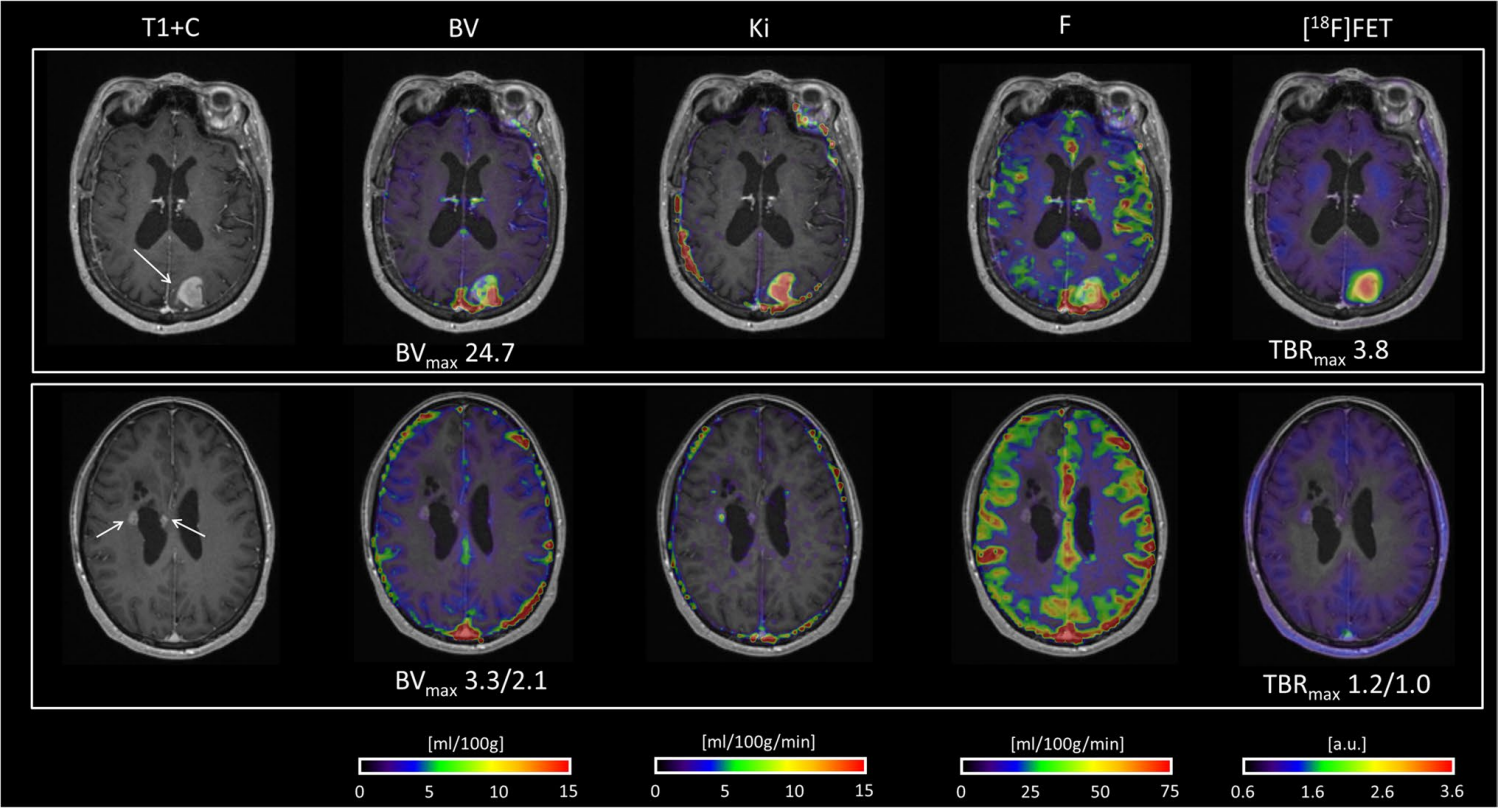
Examples of [^18^F]FET PET and DCE BV imaging providing similar information. *Upper row:* IDH-mutated GBM, progressive enhancing lesion in the left occipital lobe 7 months after surgery and radiotherapy and 1 month after completing adjuvant temozolomide. Subsequent surgery confirmed recurrent GBM. *Lower row:* IDH wild-type GBM, MRI 16 months after surgery and radiotherapy and 9 months after adjuvant temozolomide shows two stable small enhancing lesions and progressive non-enhancing signal changes (not shown) around the right lateral ventricle. PET/MRI shows increased permeability, but neither increase BV nor [^18^F]FET uptake in the two enhancing lesion. On MRI after 7 months without therapy, the lateral lesion remained stable and the medial had regressed
